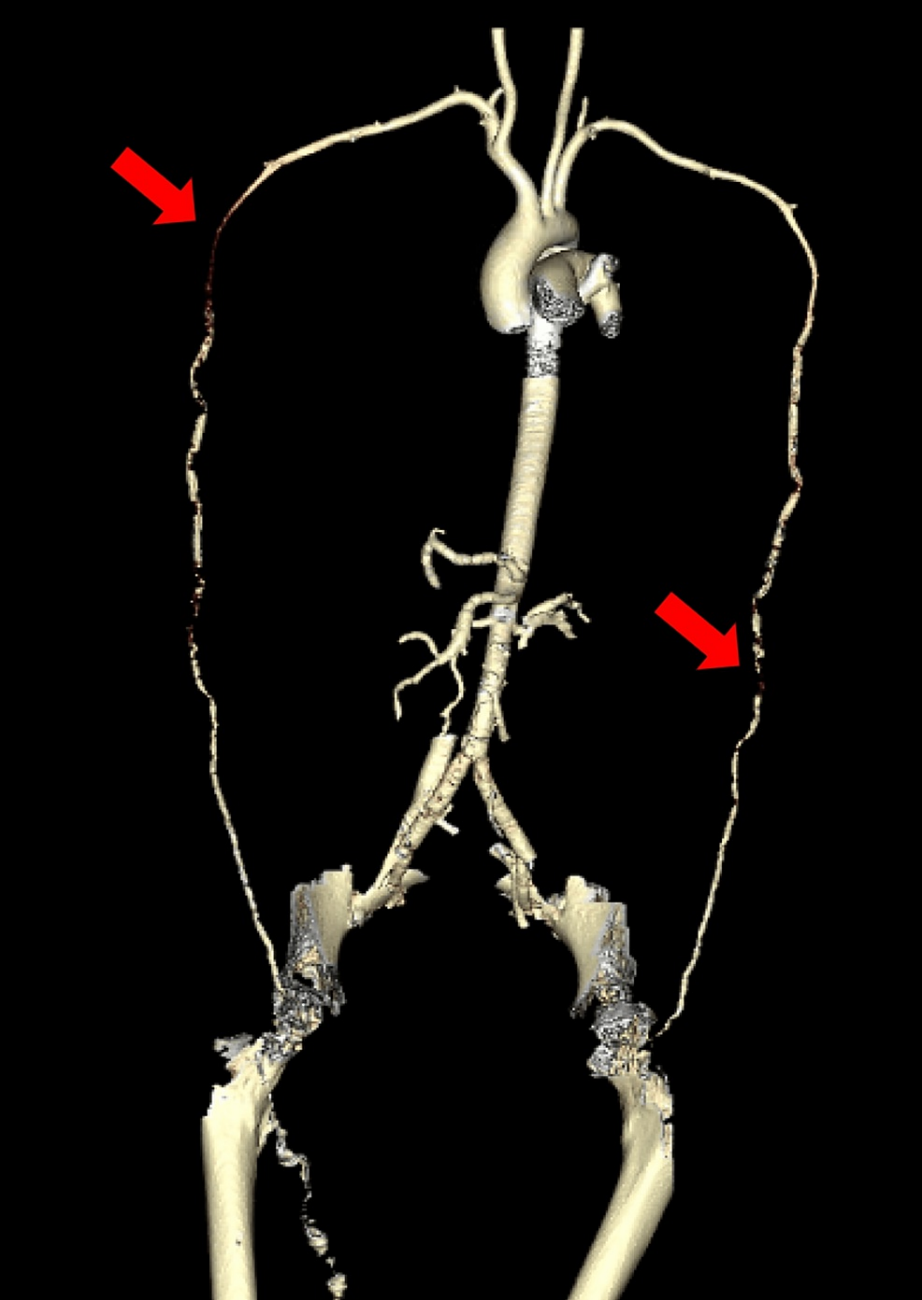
Computed tomography angiography of the chest and upper extremities with evidence of patent bilateral subclavian and axillary arteries with heavy calcification of upper extremity arteries distal to this point.
